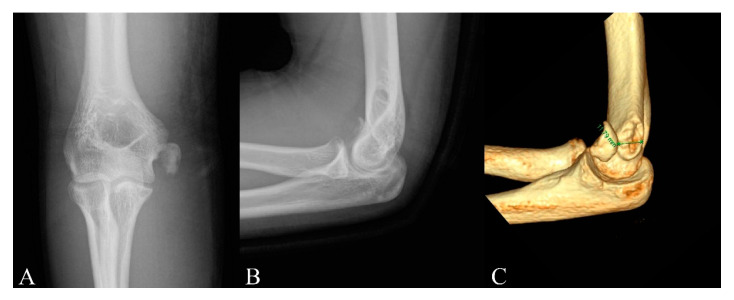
(A) Anteroposterior radiograph of a 15-year-old boy with a displaced medial epicondyle fracture. (B) Lateral radiograph demonstrates no substantial displacement of the medial epicondyle of the humerus in the same patient. (C) Three-dimensional computed tomography scans demonstrate the anterior displacement of the medial epicondyle.
